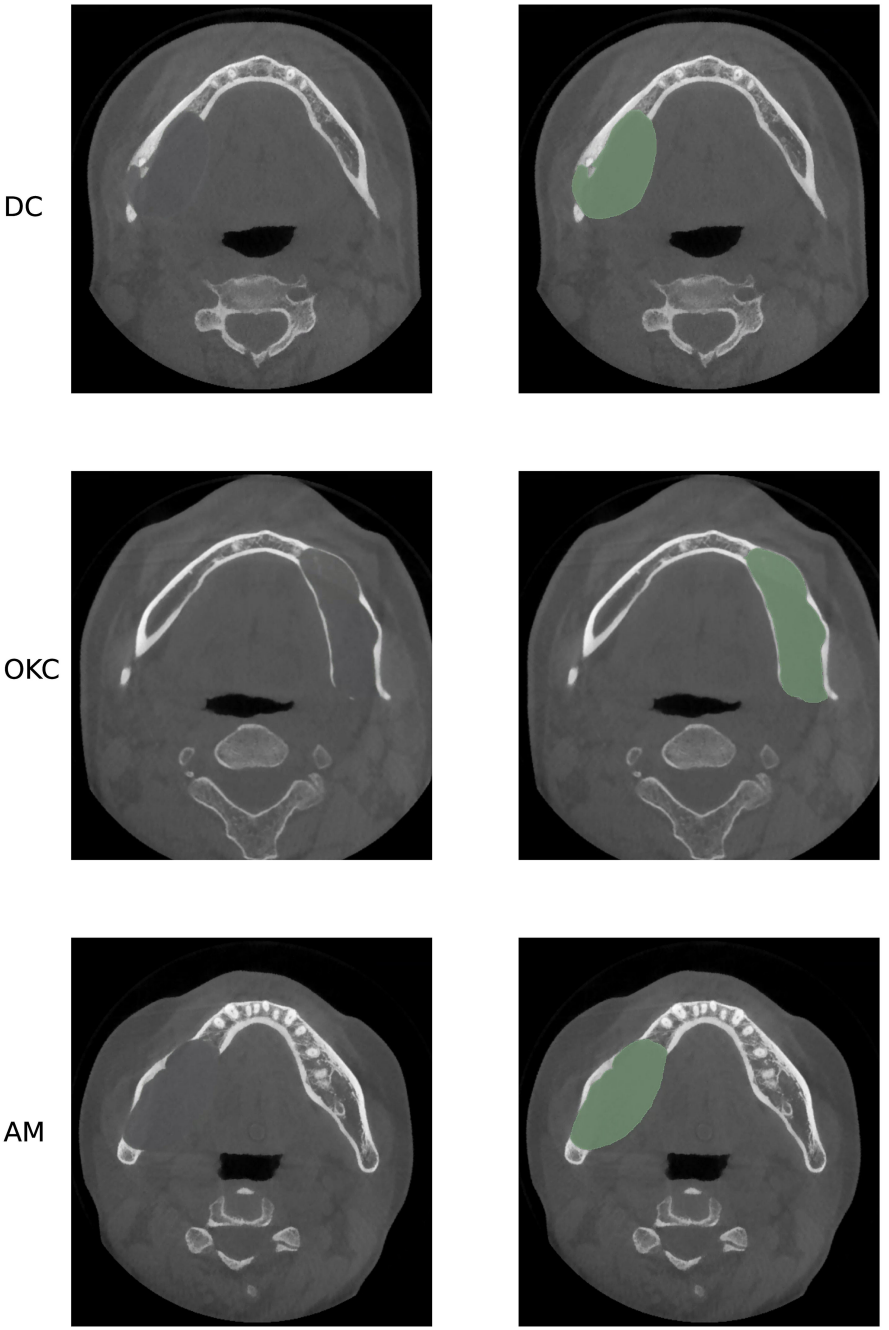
Axial CBCT presents three different lesions, all in the mandibular area, from top to bottom: DC, OKC and AM. As presented, these three lesions have similar size, location, and shape, which may cause misdiagnosis in real practice.
